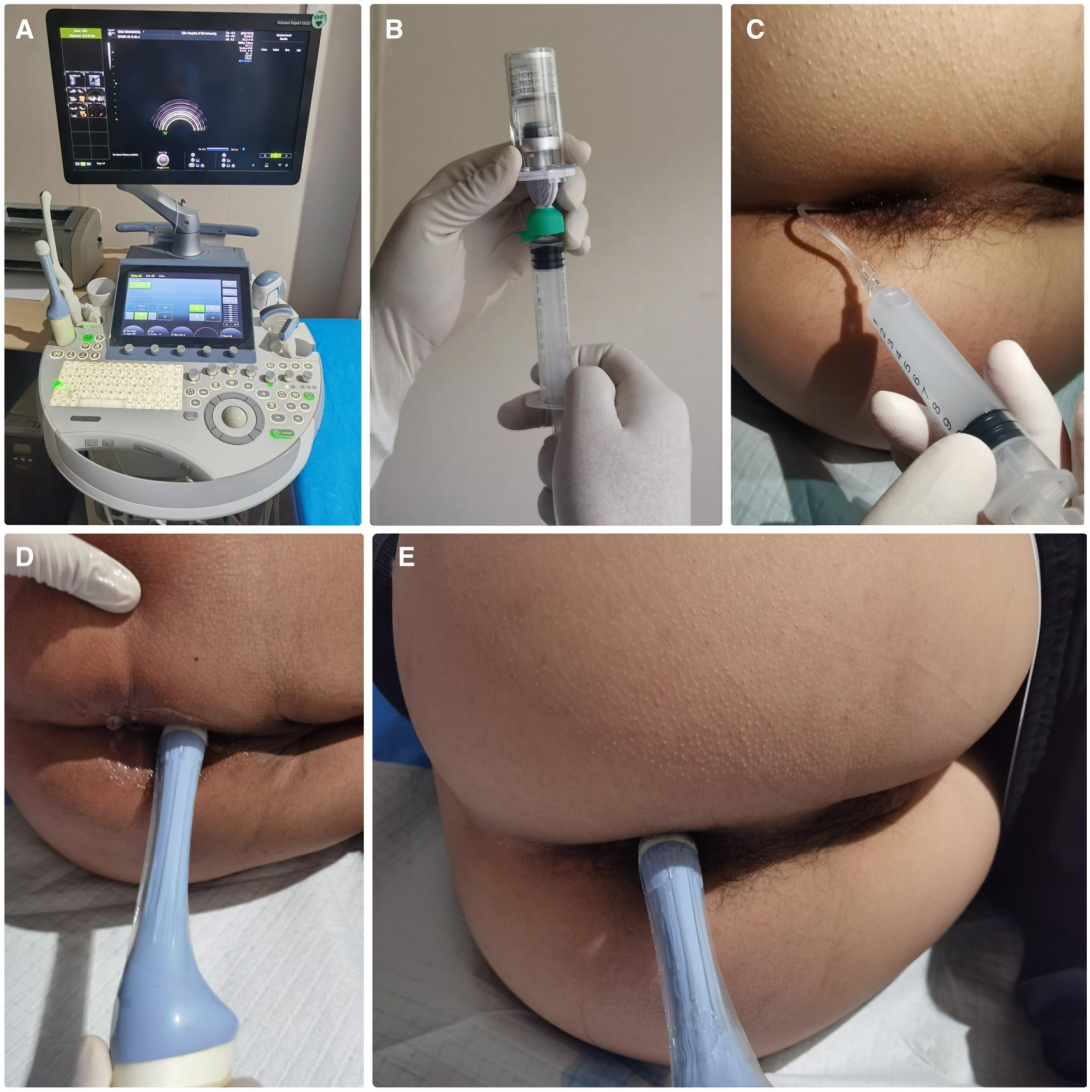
SonoVue enhancement during 3D transperineal ultrasound. (A) GE Voluson E10 diagnostic ultrasound system. (B) Proportioning SonoVue suspension. (C) Injecting SonoVue suspension. (D) Transperineal ultrasound inspection (the probe was placed above the perineal body). (E) Transperineal ultrasound inspection (the probe was placed above the anus).
